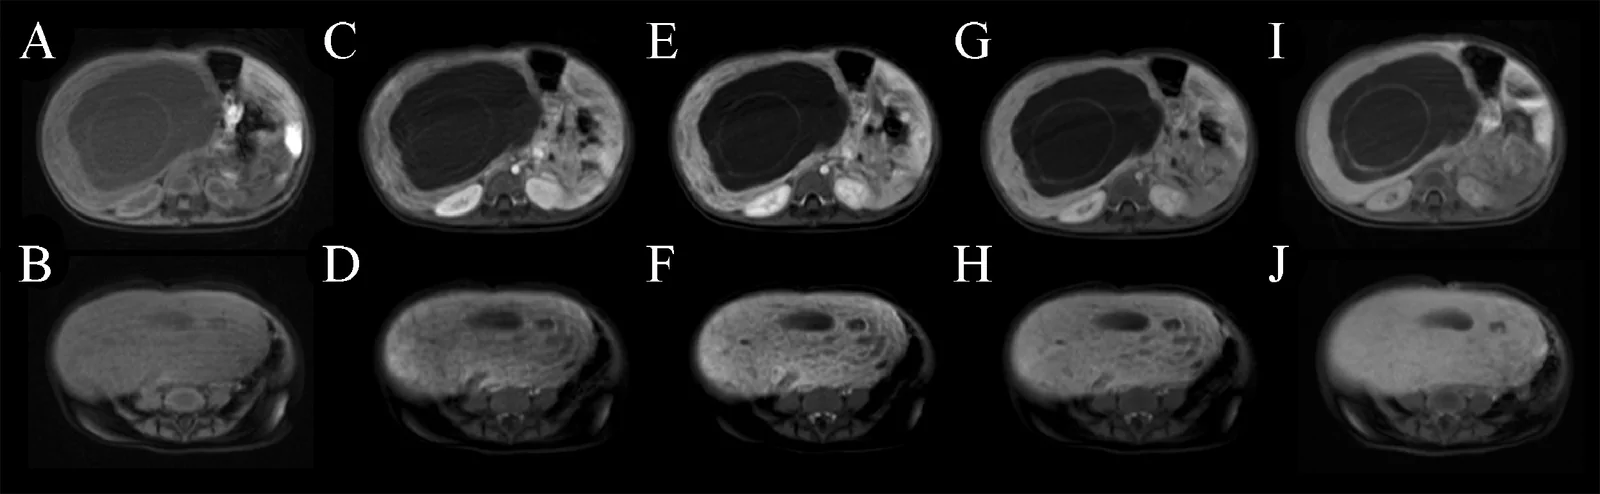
Dynamic gadoxetate disodium–enhanced magnetic resonance imaging. (A and B) Precontrast images; (C and D) 30 seconds after injection; (E and F) 70 seconds after injection; (G and H) 180 seconds after injection; and (I and J) 20 min after injection. Mild contrast enhancement was seen in the septa. The caudal region demonstrated enhancement with slightly lower signal intensity than the normal hepatic parenchyma.
